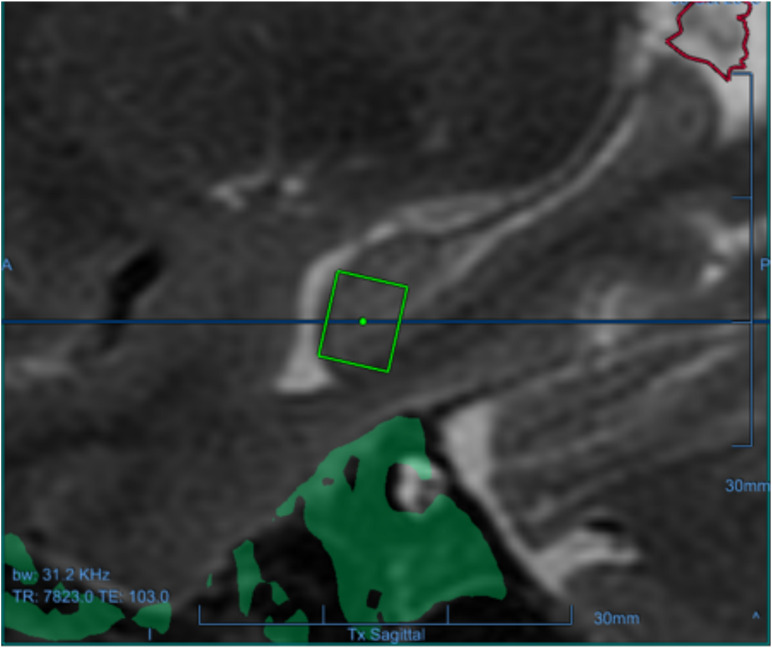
One of the targets for one of the participants as a green square in the left hippocampus, overlaid on the baseline sagittal T1-weighted MRI.
